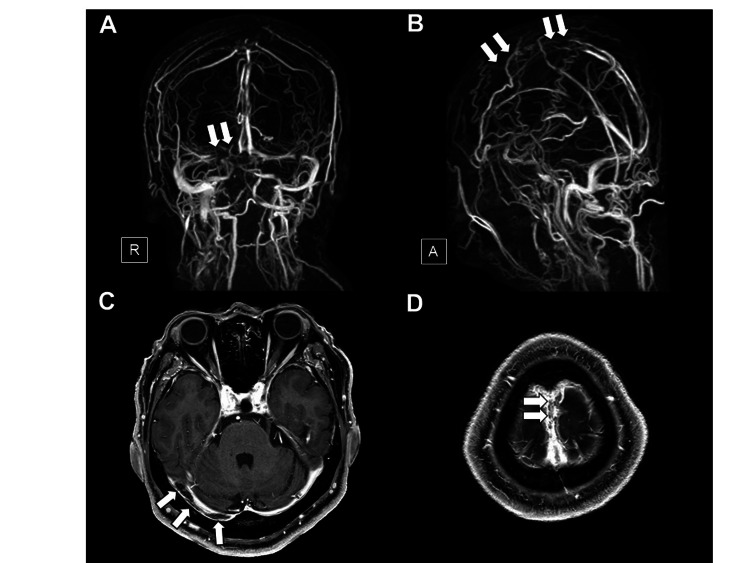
Magnetic resonance venography and brain MRI with gadolinium enhancement on admission Magnetic resonance venography (A, B) and T1-weighted brain MRI with gadolinium enhancement (C, D) on admission show thrombus as a filling defect in the right transverse sinus (A, C) (arrows) and the superior sagittal sinus (B, D) (arrows). R, right; A, anterior; MRI, magnetic resonance imaging
